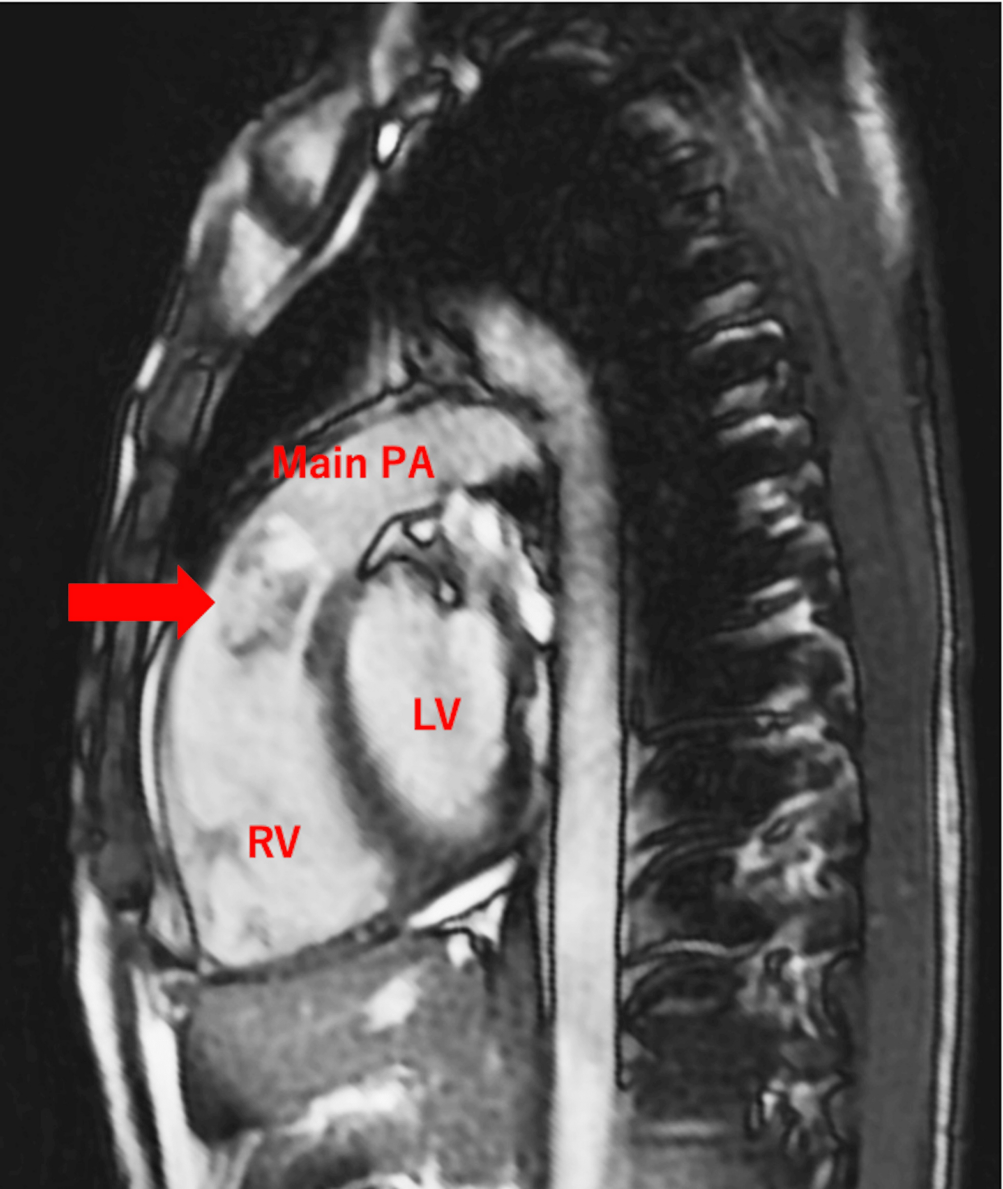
Sagittal view of the MRI showing the tumor in the right ventricular outflow tract The red arrow indicates the tumor in the right ventricular outflow tract, revealing high signal intensity within the tumor on T2-weighted imaging, reflecting the mucin contents of the tumor. MRI: magnetic resonance imaging; Main PA: main pulmonary artery; RV: right ventricle; LV: left ventricle
